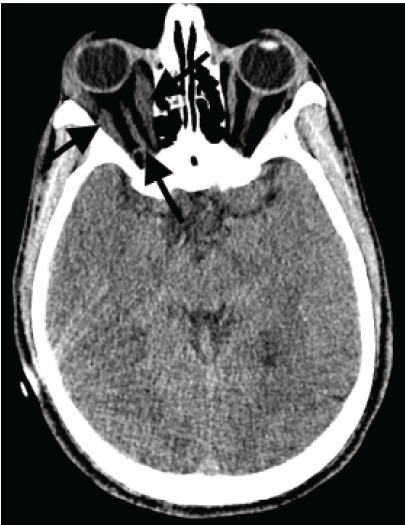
Computed tomography of the brain with thickened right extraocular muscles in a patient with orbital compartment syndrome (black arrows)
